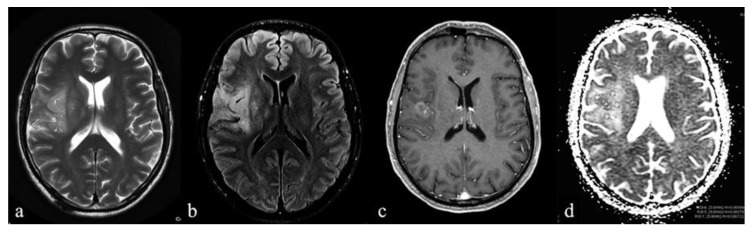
A 60-year-old man with an anaplastic astrocytoma IDH-Wt. (a,b) Axial T2-weighted and FLAIR images demonstrate a lesion with high signal intensity and indistinct borders on the right insular lobe; contrast-enhanced axial T1-weighted image (c) demonstrates a blurred contrast-enhancement within the lesion corresponding to the lower mean ADC (ADCm) value (d). Scale bar: 5 cm.
